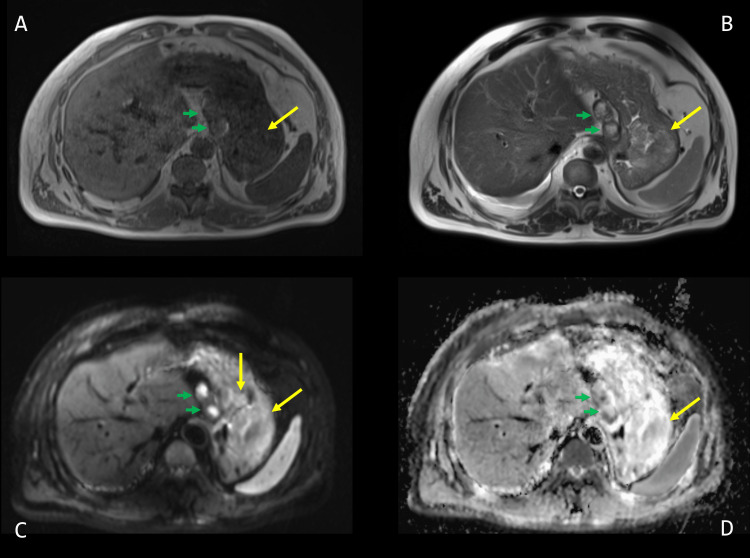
Axial T1-weighted (A), T2-weighted (B), and diffusion-weighted (C) magnetic resonance imaging of the abdomen with apparent diffusion coefficient (D) Two gastric mucosal lesions (yellow arrows) appear as polypoid masses protruding into the lumen of the gastric fundus and body. They appear hypointense on the T1-weighted image (A) and slightly hyperintense on the T2-weighted image (B). They show no significant diffusion restriction on diffusion-weighted imaging (C) and apparent diffusion coefficient (D). In addition, two necrotic adenopathies (green arrows) are visible against the lesser curvature of the stomach.
